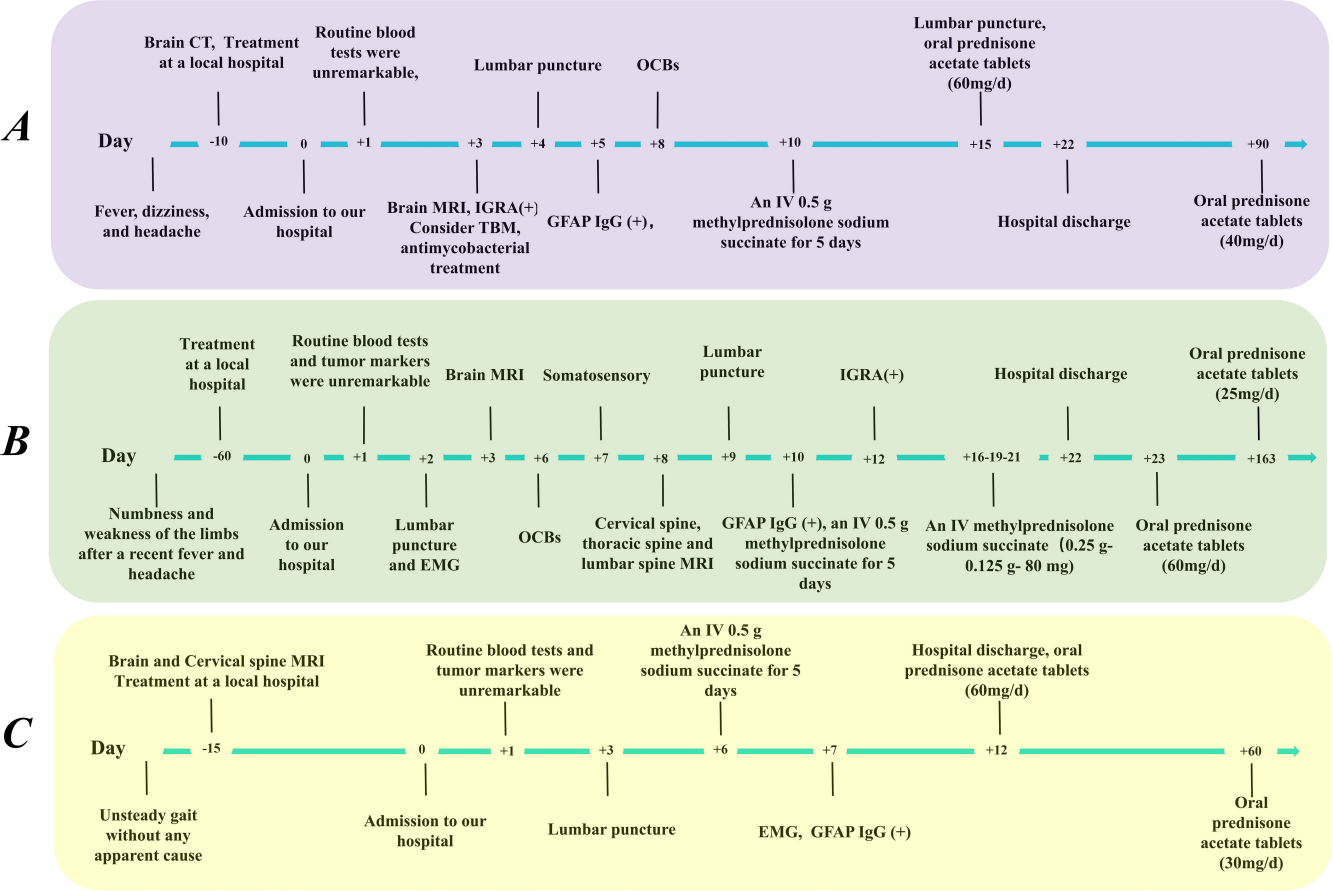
Timeline of clinical manifestations and treatment progression in our patients. (A) Patient A, (B) Patient B, (C) Patient C. CSF, cerebrospinal fluid; CT, computed tomography; EMG, electromyography; IGRA, interferon gamma release assay; IV, intravenous; GFAP, glial fibrillary acidic protein; MRI, magnetic resonance imaging; OCBs, oligoclonal bands.
